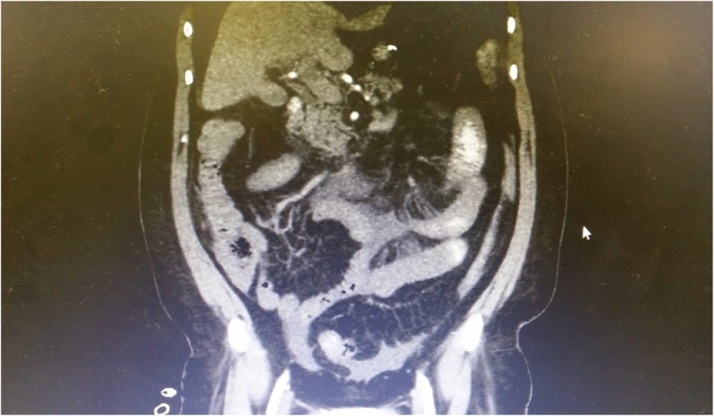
Computed tomography (CT) of abdomen showing porto-mesenteric vein thrombosis with evidence of small bowel ischemia.
